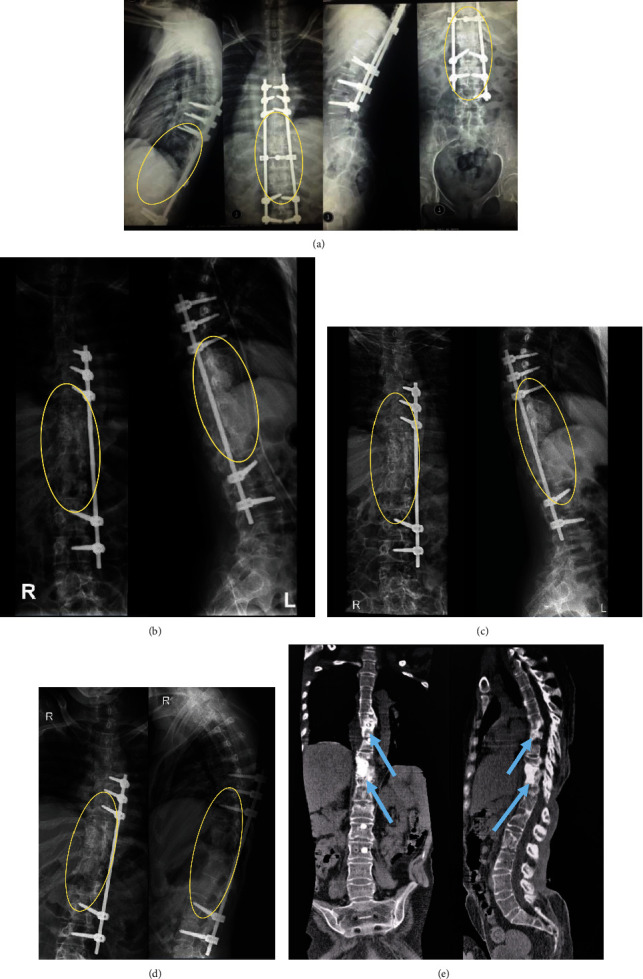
A 30-year-old female (case 3). (a) Preoperative radiography showed defects on multiple corpus vertebrae (T9-12). (b) One-month postoperative radiography showed around 50% of new bone formation along T9-L1, and postoperative radiography also showed instrumentation planted on the left side. (c) Three-month postoperative radiography showed around 65% of new bone formation. (d) Six-month postoperative radiography showed around 85% of new bone formation. (e) Six-month postoperative CT scan showed around 85% of new bone formation. Sites are within yellow circles and pointed using white, black, and blue arrows.
